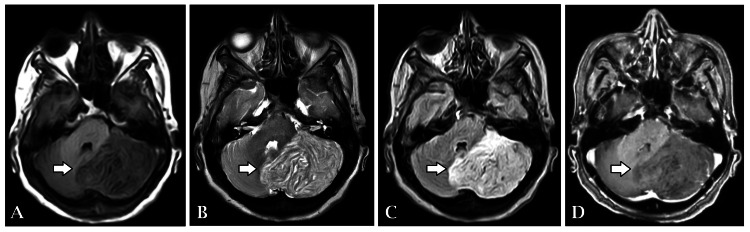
(A, B, C) The left cerebellar hemisphere was enlarged with altered signal intensity, presenting a tigroid appearance on T1-weighted, T2-weighted, and FLAIR images. (D) There was no evidence of enhancement post-contrast FLAIR: fluid-attenuated inversion recovery
